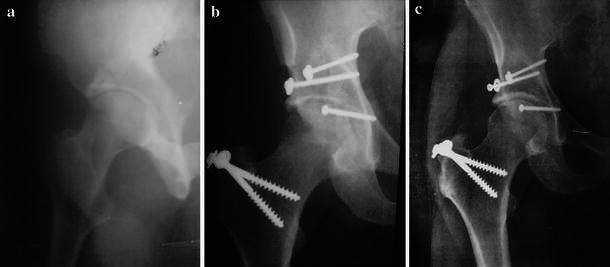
a Preoperative anteroposterior (AP) radiographs of a 27-year-old man showing posterior acetabular-wall fracture, b osteosynthesis of the posterior wall using lag screws and trochanteric flip osteotomy; postoperative AP radiographs showing anatomical reduction, c AP radiograph at 10-years’ follow-up showing excellent radiological outcome
